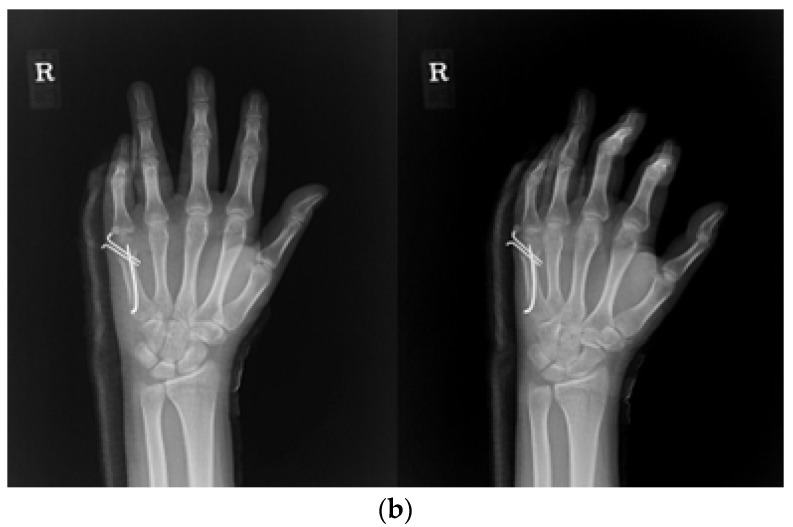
(a) Radiograph of an 18-year-old male patient with displaced little-finger metacarpal neck fracture. (b) Radiograph of a 16-year-old male patient who underwent the IPKP method using one intramedullary nail and two additional K-wires.
